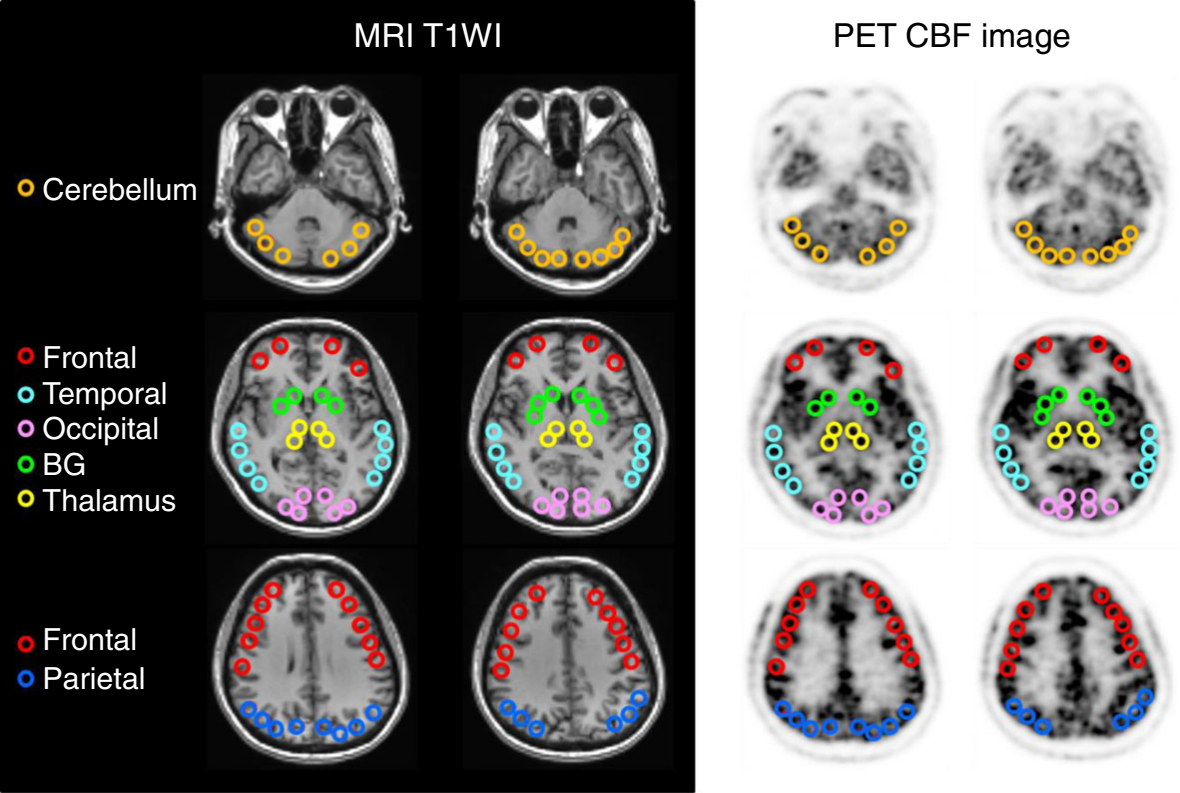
ROIs on several representative slices of MRI (left two columns) and PET (right two columns). Different colors shows different locations of frontal (red), temporal (cyan), occipital (light pink), parietal (blue) lobes, basal ganglia (green), thalamus (yellow), and cerebellum (orange). ROIs were placed in the MRI images and transferred to the CBF-PET in the same location
